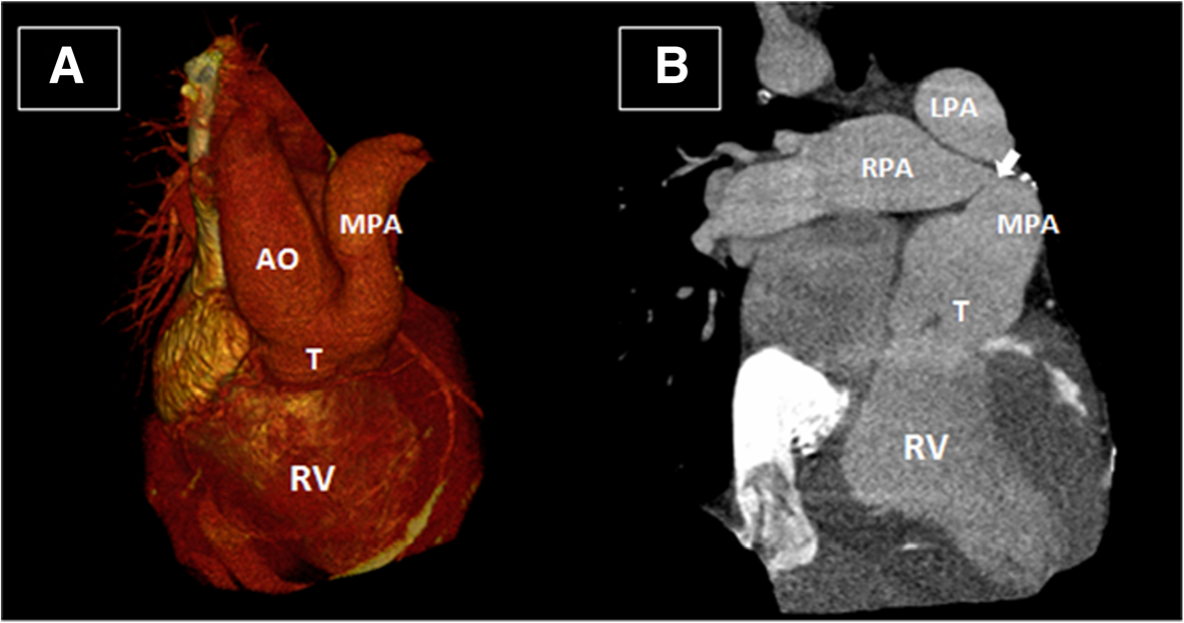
Computed tomography of the chest. The heart size is enlarged. On the 3-dimensional volume rendered image (a), there is a common trunk of the ascending aorta and main pulmonary artery (PA). The aortic arch is right-sided and the pulmonary arteries are grossly dilated. On the coronal view (b), the tight narrowing at the beginning of right PA (white arrow) is where the migrated PA band from main PA locates. T: truncus; MPA: main pulmonary artery; LPA: left pulmonary artery; RPA: right pulmonary artery; AO: aorta
